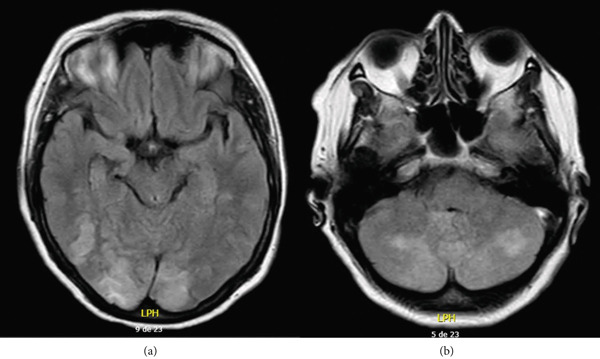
Hyperintense lesions in the occipital and cerebellar lobes. Note: axial slices from a brain magnetic resonance imaging in fluid‐attenuated inversion recovery sequence showing hyperintense corticosubcortical lesions, bilaterally located in the (a) occipital and (b) posterior cerebellar lobes.
